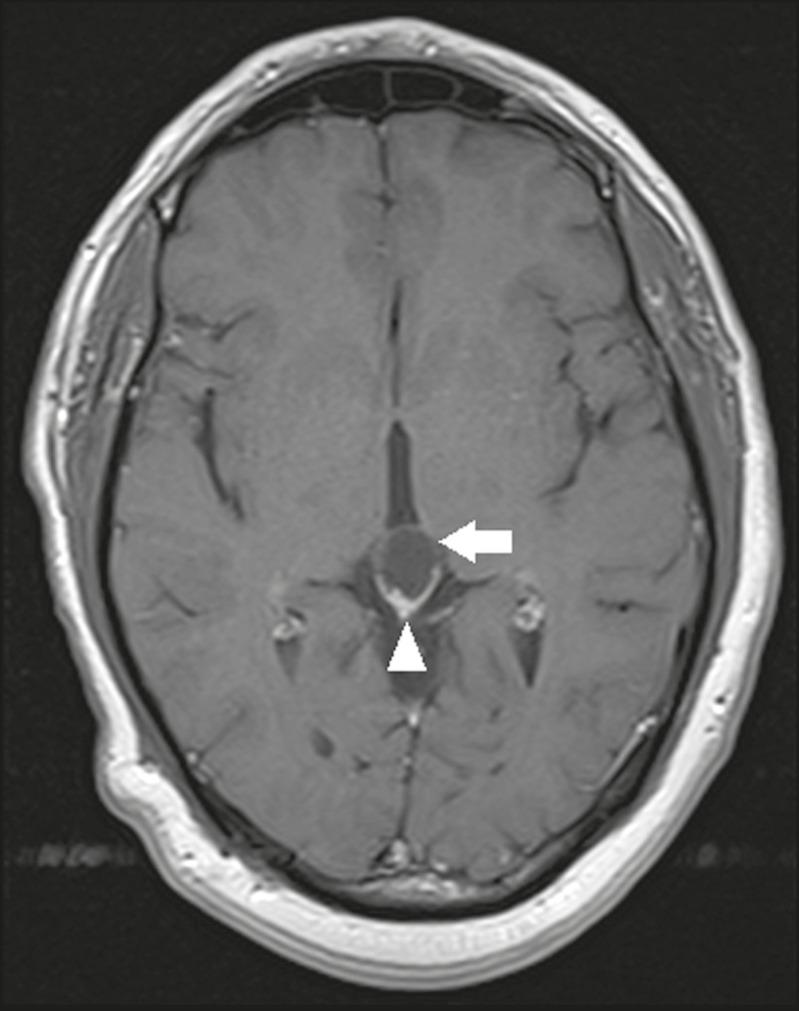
Pineal cyst. Contrast-enhanced axial T1-weighted MRI showing a cyst-like formation in the pineal gland region, with no evidence of septations or mural nodules (arrow), with a thin, smooth line of contrast enhancement along the margin (arrowhead).
